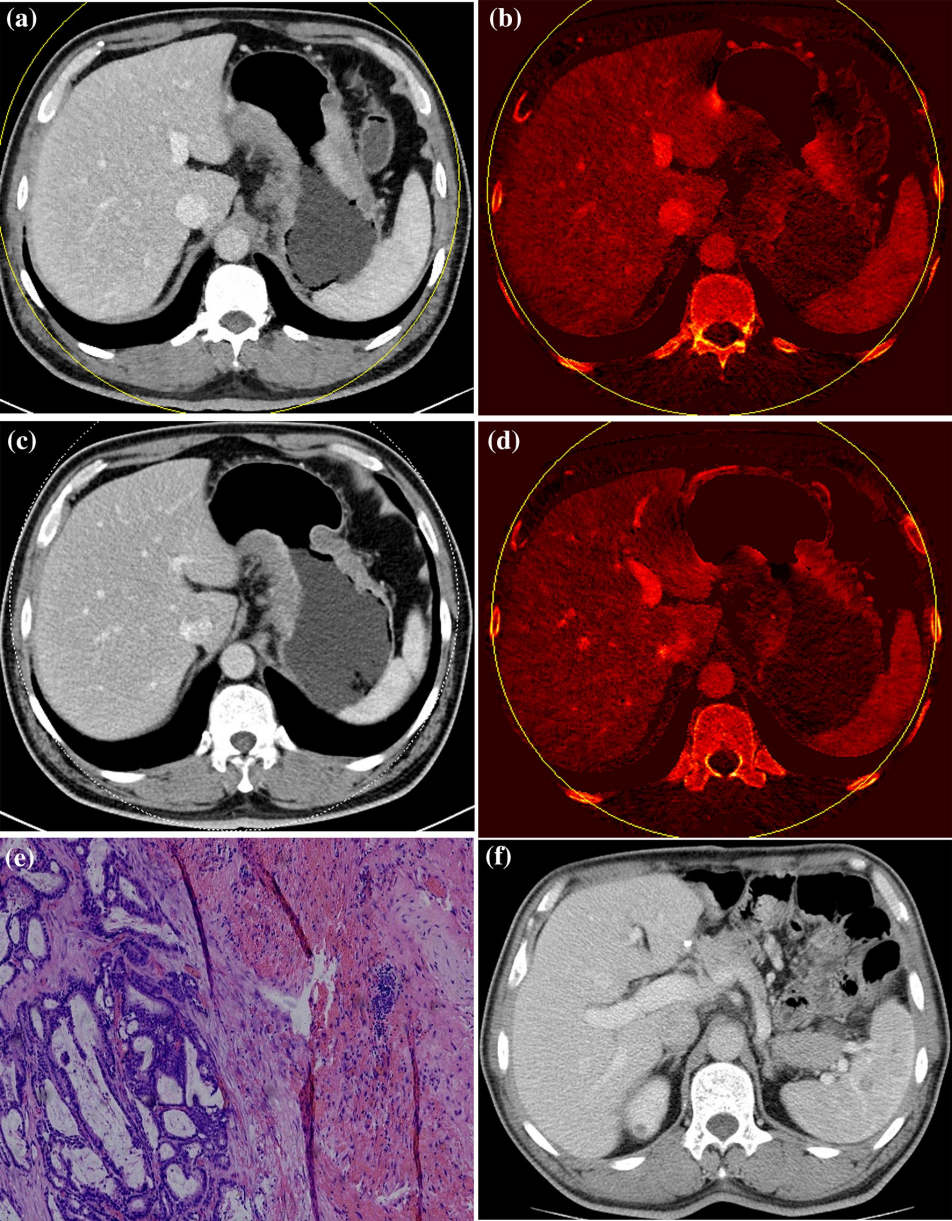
Baseline CT image (a, b) and follow-up CT image (c, d) of a patient (male, 51 years) after three cycles of chemotherapy (%ΔS: − 21.44%, %ΔD: − 26.88%, %ΔHU: − 14.66%, %ΔV: − 39.74%, %ΔTIU-p: − 60.09%). According to the Choi criteria, this patient was classified as a responder, but according to other criteria, he was classified as a non-responder. Finally, the pathology of this patient (e) was grade 2, minimal tumor regression (40% residual tumor per tumor bed with intratumoral hemorrhage). Furthermore, this patient was found to have spleen metastasis after 15 months (f)
